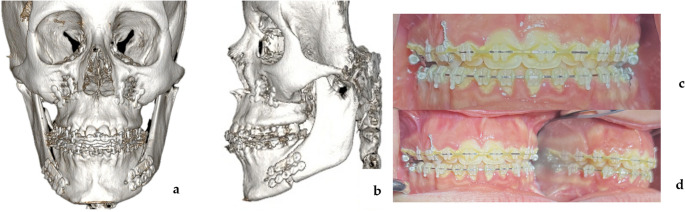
Post-operative images of Case 2 (a) post-operative frontal view CT scan (b) post-operative lateral view CT scan (c) final occlusion in frontal view (d) final left lateral occlusion
